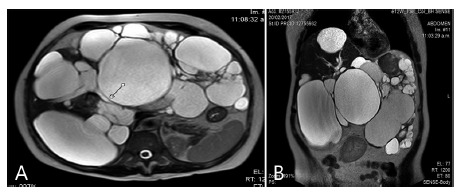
MRI showing severe APLD compressing hepatic pedicle and occupying almost whole abdominal cavity
